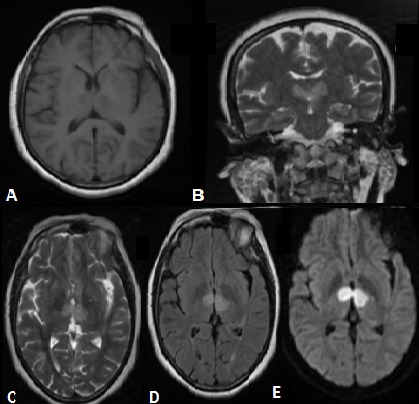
IRM cérébrale en séquences axiales T1 (A),T2 (C), T2 FLAIR(D), diffusion b1000(E) et coronale T2(B): anomalies de signal des deux noyaux thalamiques en hyposignal T1, hypersignal T2 et T2 FLAIR avec restriction de la diffusion en rapport avec un accident vasculaire cérébral ischémique récent bithalamique par occlusion de l'artère de Percheron
